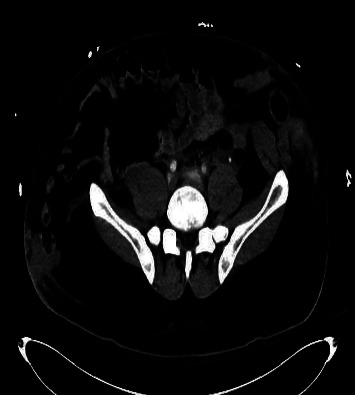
Abdominal CT. Bilateral herniation of abdominal viscera.
